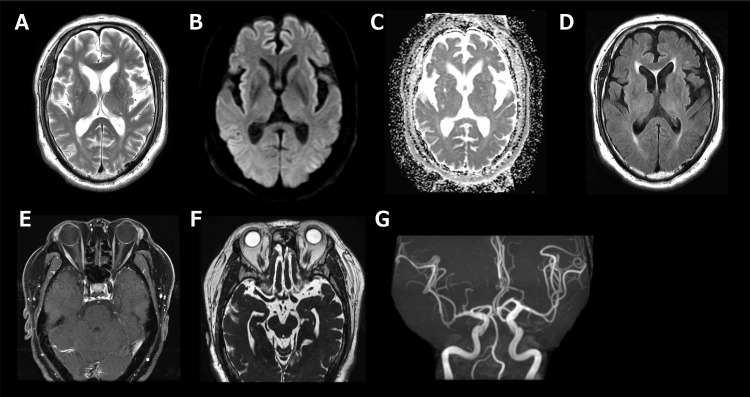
Brain MRI at admission. T2-weighted imaging (A), diffusion-weighted imaging (B), apparent diffusion coefficient (ADC) map (C), fluid-attenuated inversion recovery (FLAIR) (D), contrast-enhanced MRI (E), 3D-FIESTA (fast imaging employing steady-state acquisition) (F), and magnetic resonance angiography (G) revealed no evidence of an aneurysm or other abnormalities.
